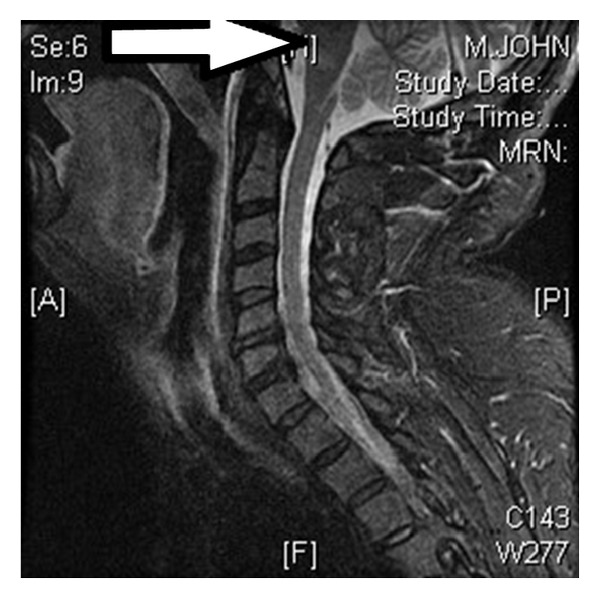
MRI of cervical spine, sagittal, shows abnormal T2 hyperintense lesion at mid-upper medulla.
